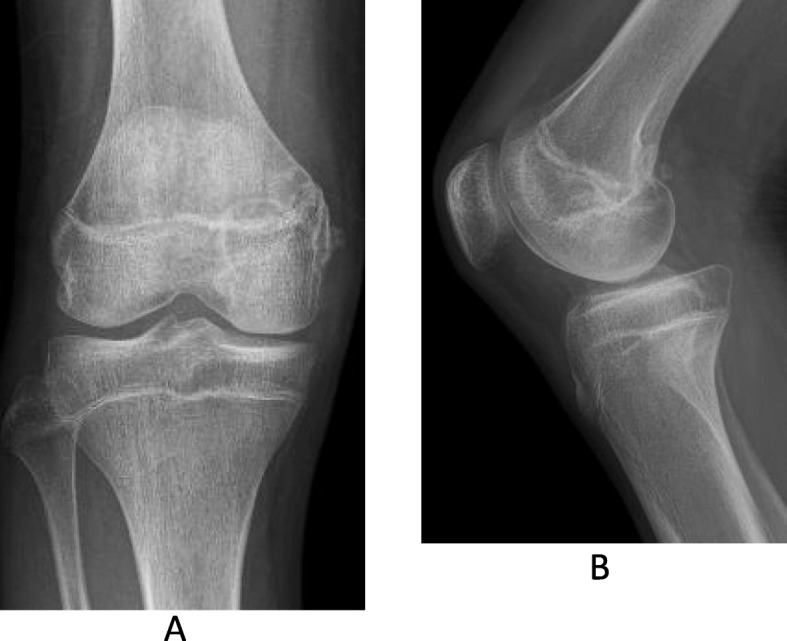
a and b Plain radiographs of the injured knee 3 months after surgery. Anteroposterior (a) and lateral (b) radiographs showing solid osseous healing of the avulsion fracture of the medial collateral ligament and the avulsion fracture of the medial head of the gastrocnemius muscle
